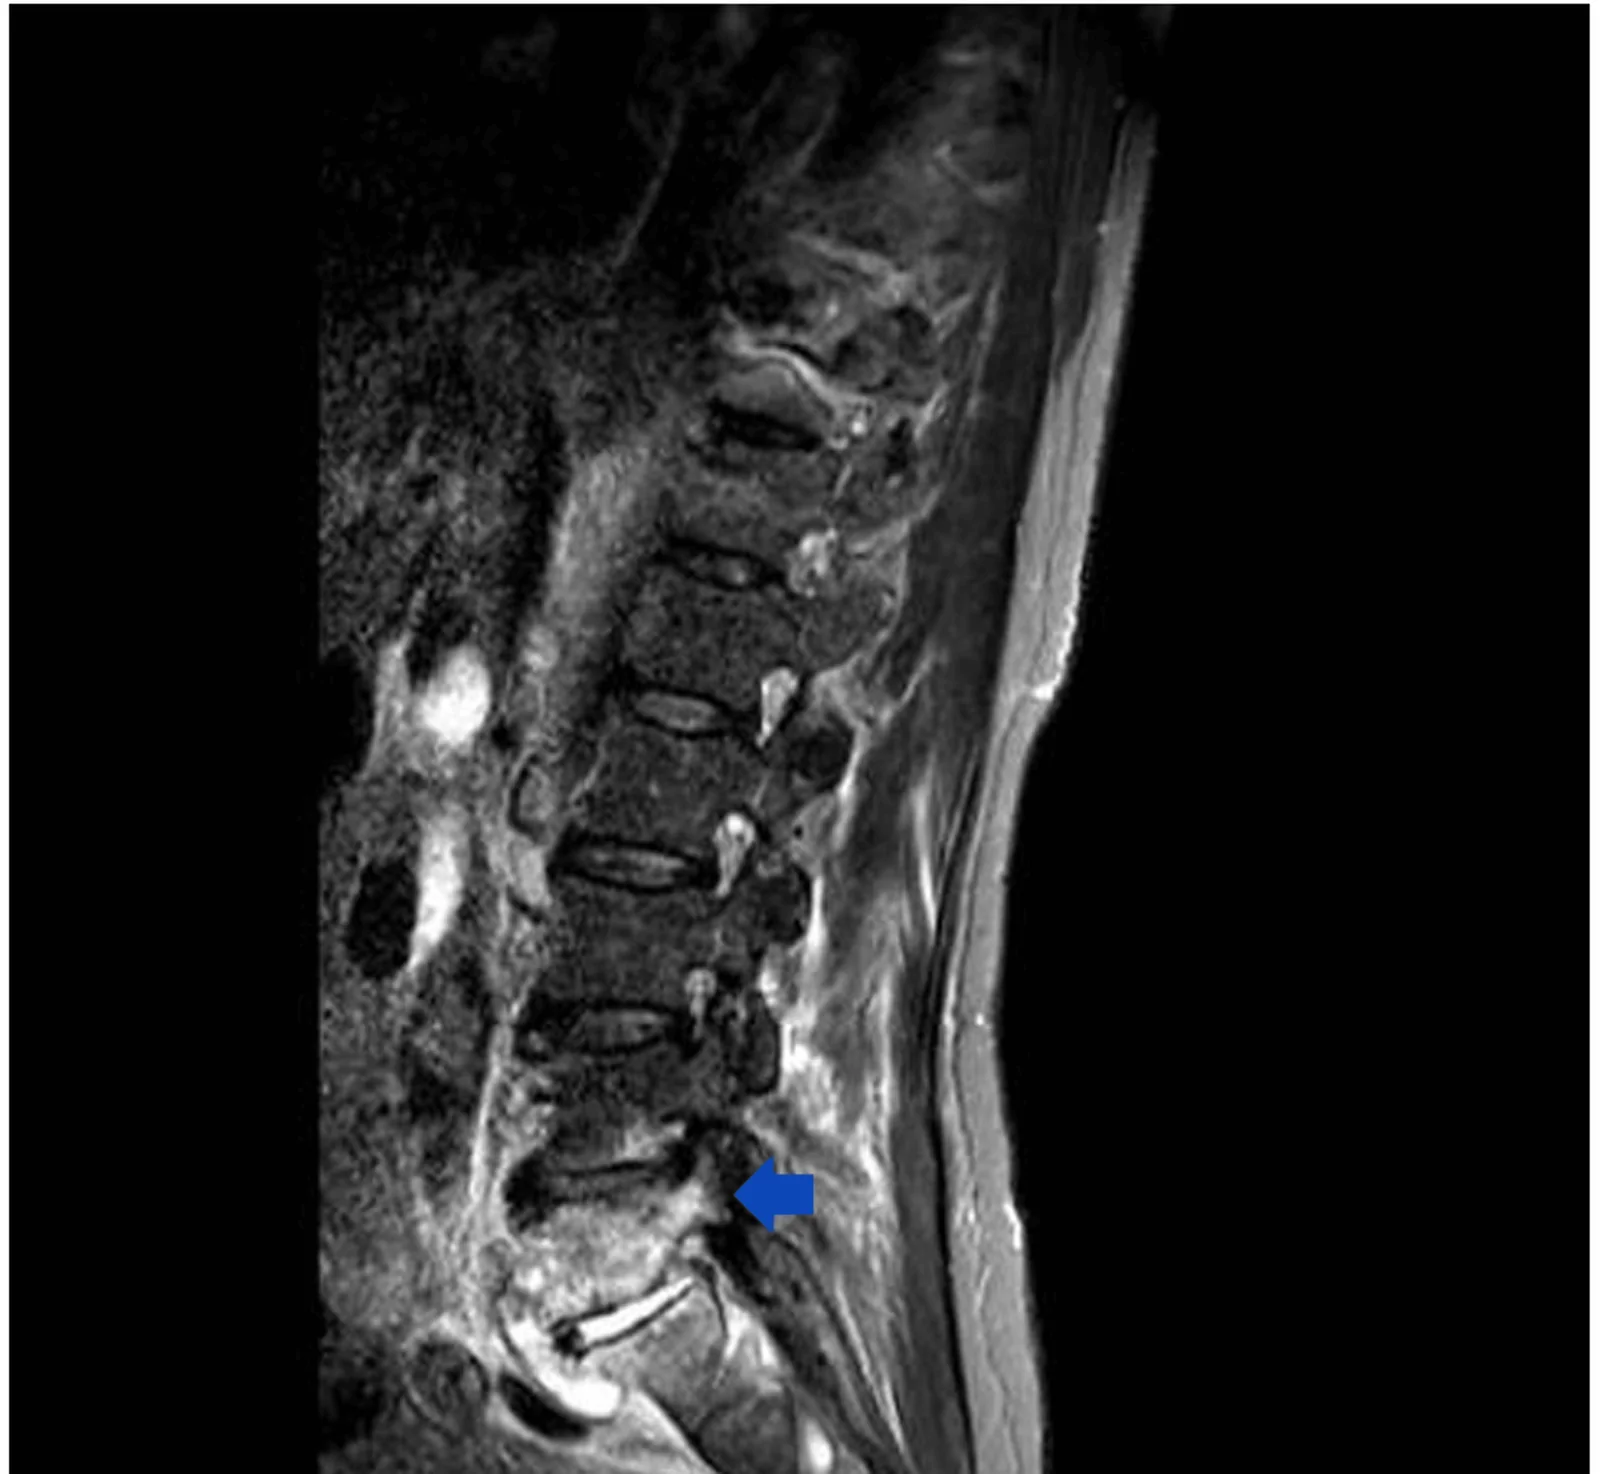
Repeat whole-spine MRI demonstrating fifth lumbar vertebra–first sacral vertebra (L5–S1) spondylodiscitis complicated by an anterior epidural abscess causing cauda equina compression, with associated anterior prevertebral fluid collection (blue arrow).
